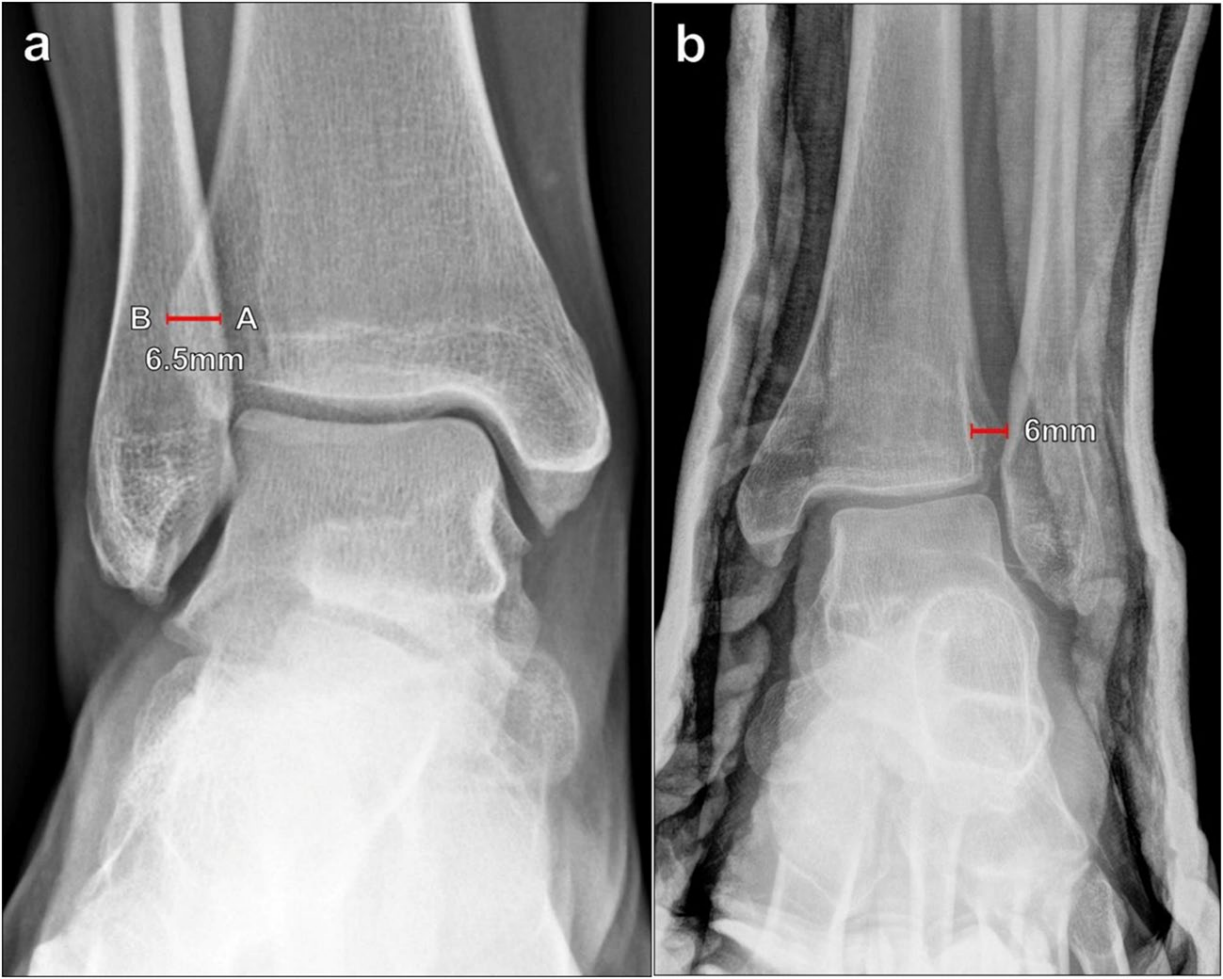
Measurement of tibiofibular overlap on AP radiograph of the ankle. a The horizontal distance between the medial margin of the distal fibula (A) and the lateral margin of the tibial tubercle (B) is measured 1 cm proximal to the tibial plafond. The tibiofibular overlap should be > 6 mm on the AP view and > 1 mm on the mortise view. The absence of tibiofibular overlap in conjunction with tibiofibular and medial tibiotalar diastasis suggests syndesmotic instability. b Absent tibiofibular overlap on AP radiograph of the ankle on the same patient seen on Fig. 5b, indicating syndesmotic insufficiency. Even on the AP radiograph, the tibiofibular clear space measures 6 mm, which also indicates syndesmotic disruption
